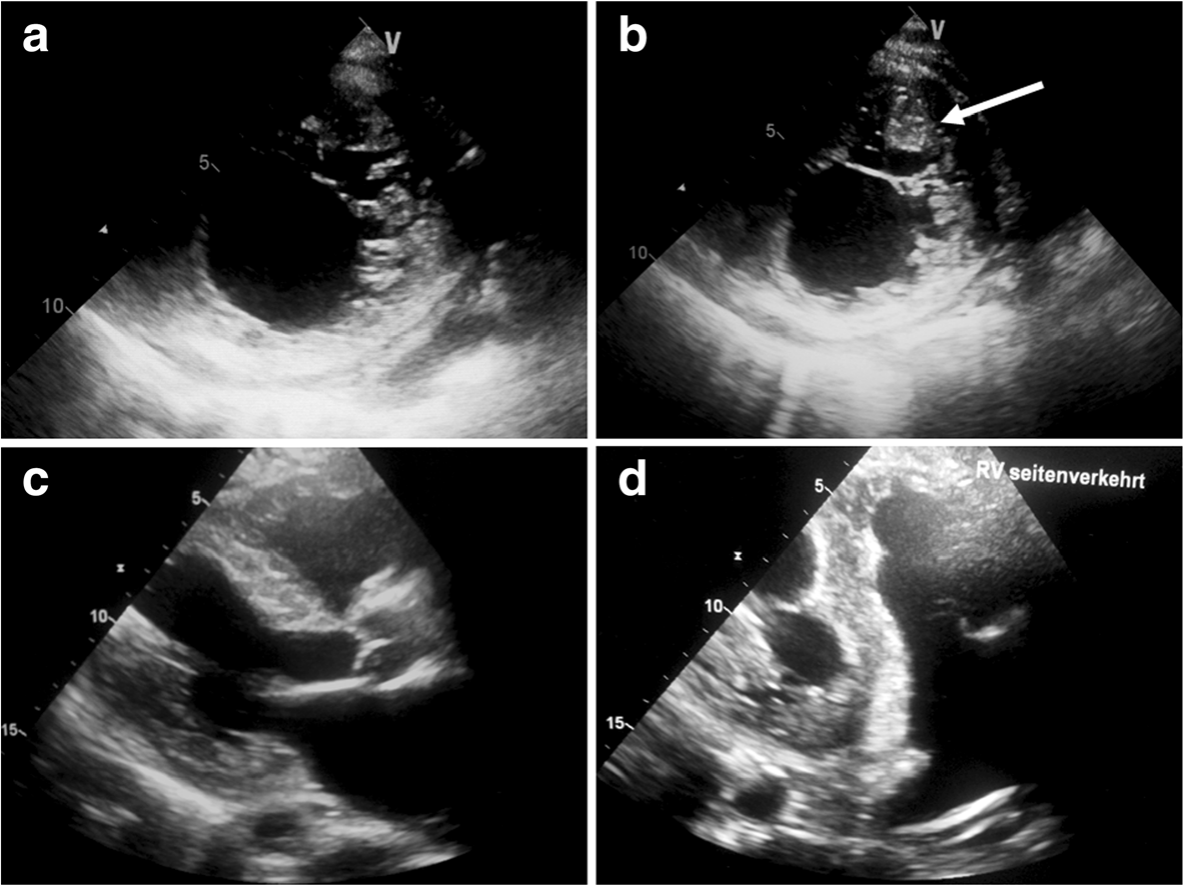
Transthoracic echocardiography 9 months after LVAD implantation: a short axis view showing trabeculated left ventricular myocardium; b tilted short axis view showing a solid structure stretching across the LV in front of the LVAD inflow cannula (arrow), c parasternal long axis view showing thick, spongy LV myocardium and narrow LV cavity, d inverted tilted four chamber view showing thickened LV myocardium with small LV cavity appearing divided by a solid structure
